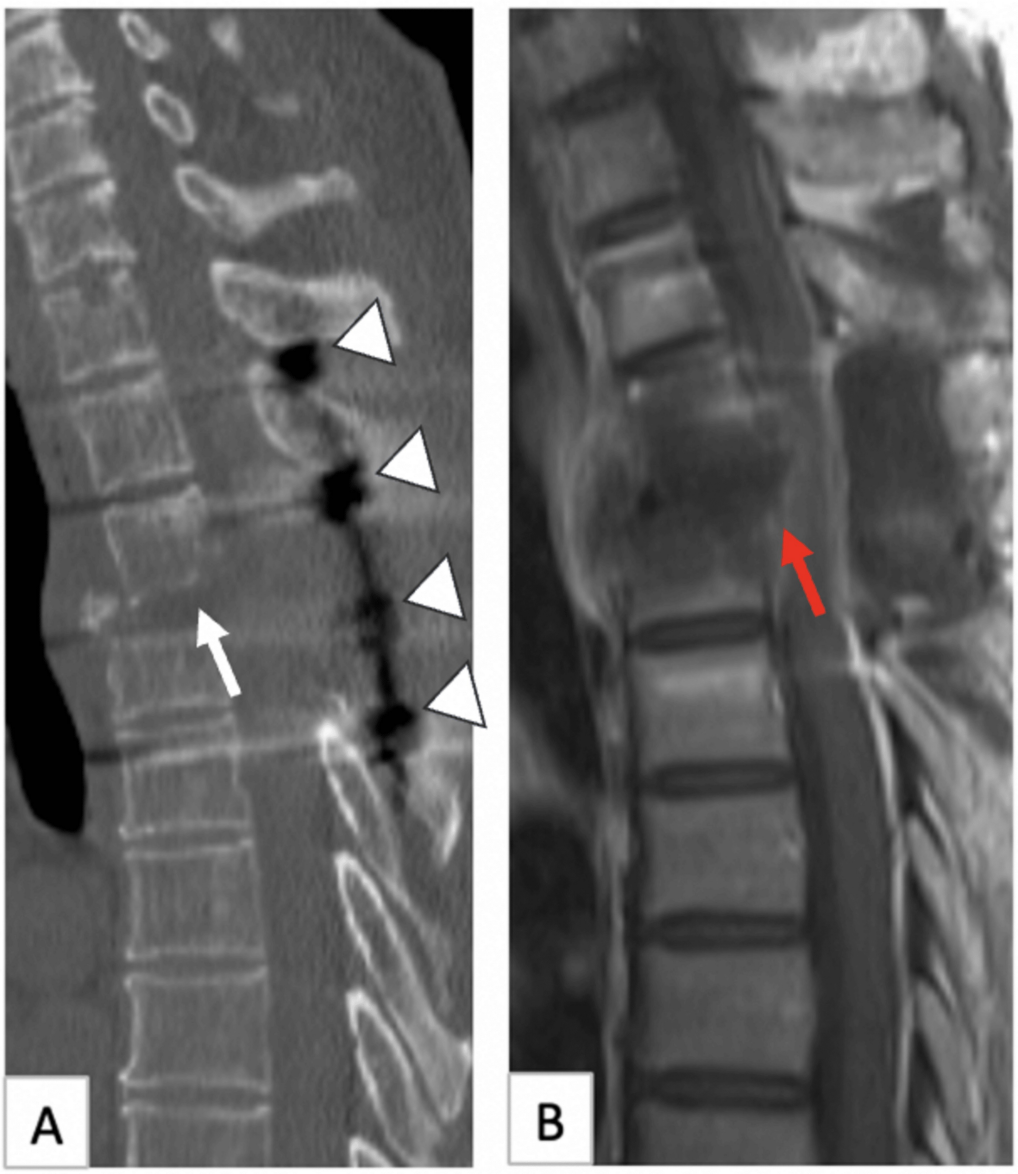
Postoperative images of the patient A: Sagittal CT shows that the fourth thoracic vertebra with compression fracture was removed by corpectomy (white arrow) and instrumented (arrowhead). B: Sagittal post-contrast MR sequence demonstrates reduction of edema and resection of the mass (red arrow).
